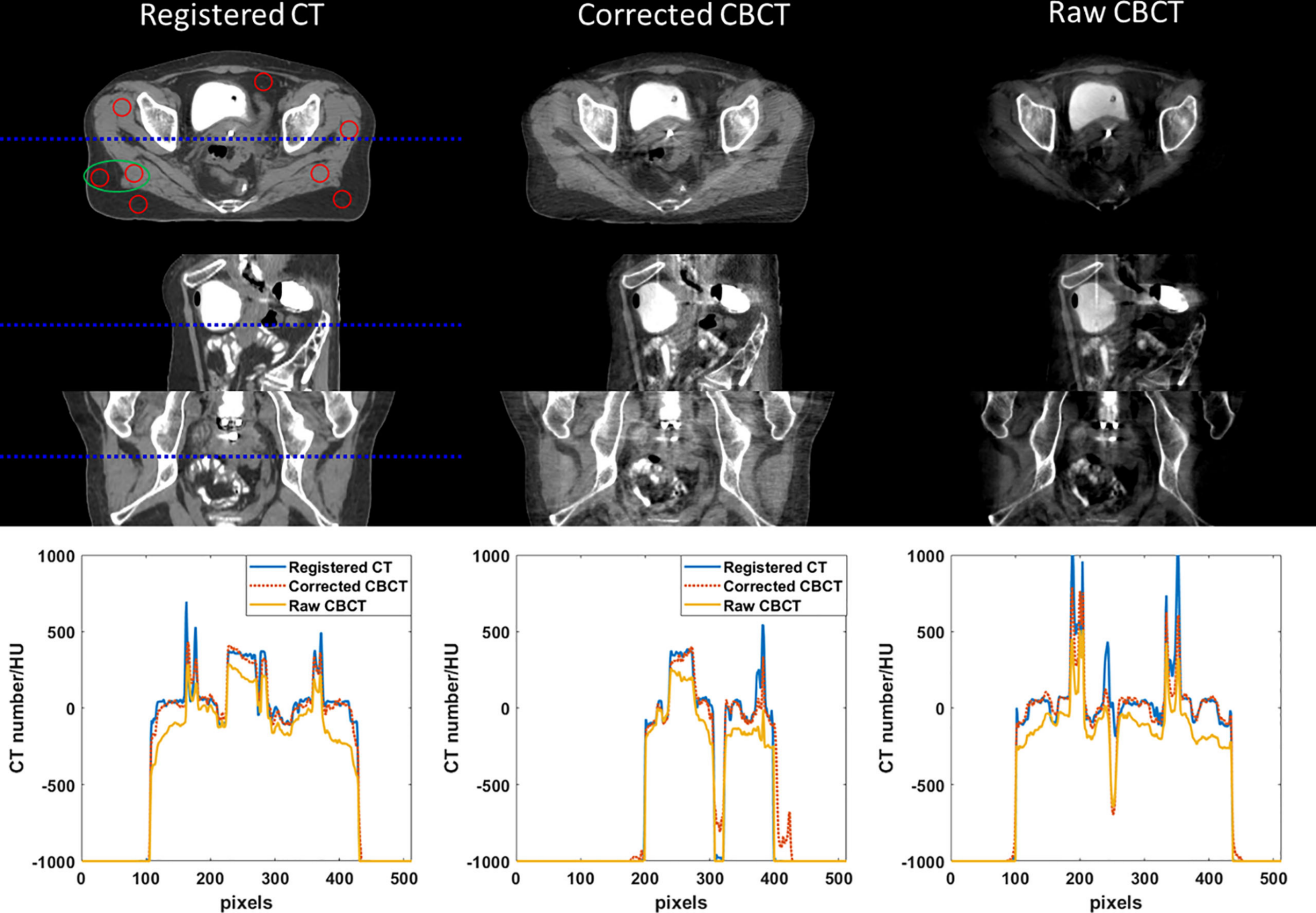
CT/CBCT images of one BT patient. The first three rows display the coronal, sagittal, and axial views from top to bottom; display window: [−200 300] HU. The 1D profiles indicated by the dotted lines are displayed in the last row. The mean CT number and SNU are calculated in the circle areas, and the CNR is calculated in the ellipse area.
